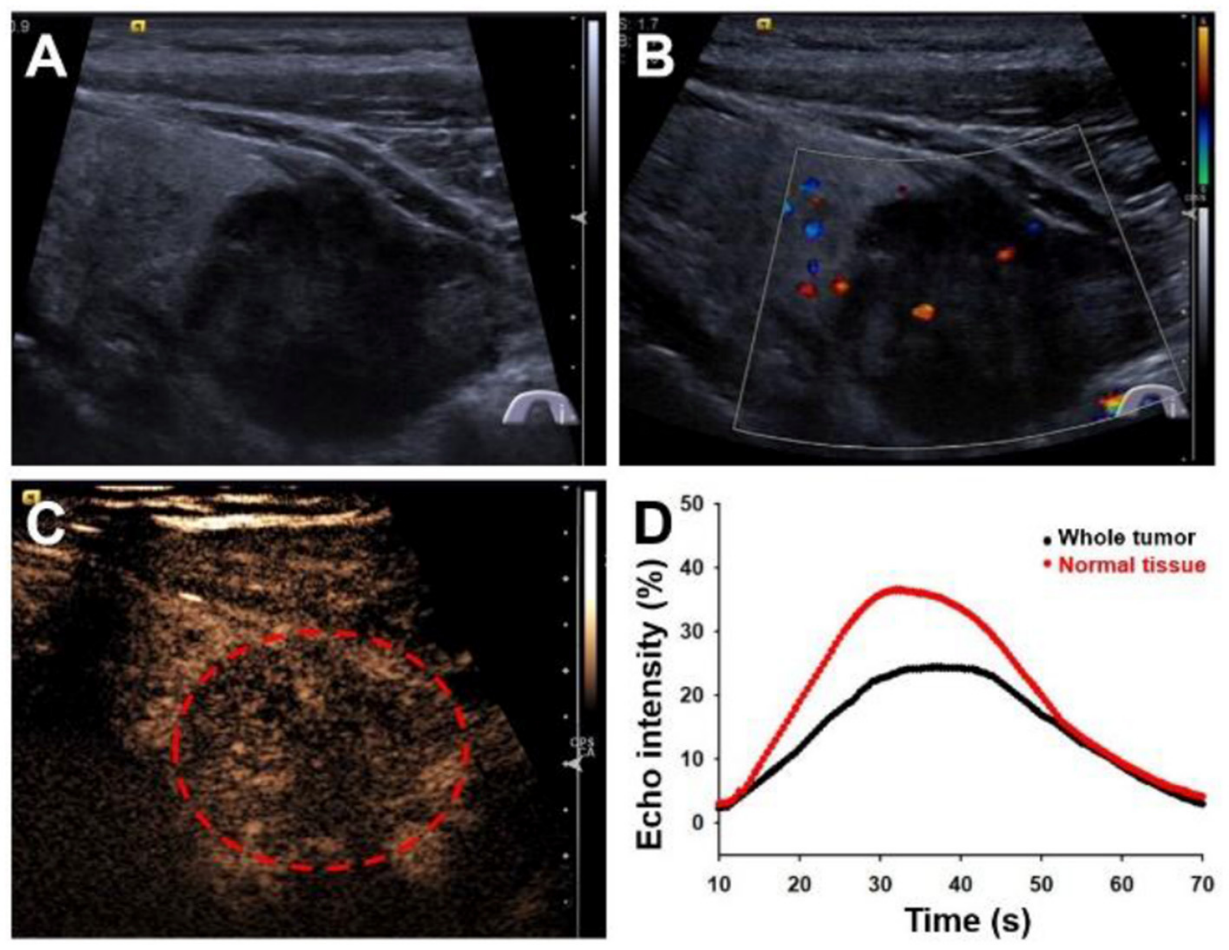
Ultrasonography images of primary squamous cell carcinoma of the thyroid. (A) Longitudinal gray-scale sonography revealed a solid marked hypoechoic thyroid nodule in the inferior part of the left lobe. (B) Color Doppler flow imaging showed a poor blood flow signal inside this nodule. (C) Contrast-enhanced ultrasound image showed a persistent low peak enhancement of the nodule at 37 s. (D) Time-intensity curves displayed the wash-in time of 10 s, TTP of 37 s, peak signal intensity of 24.5%, and wash-out time of 70 s for the thyroid tumor.
